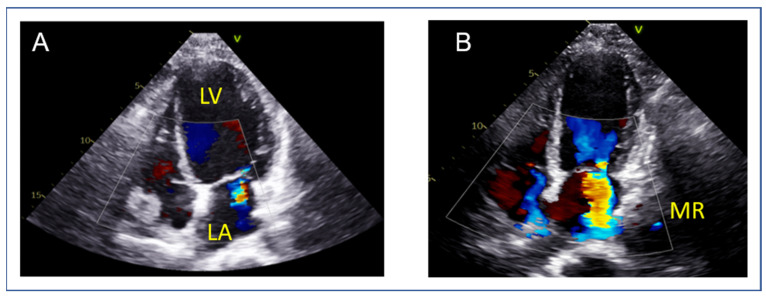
Ischemic dynamic MR unmasked by exercise echocardiography in a 66 y.o. patient with ischemic cardiomyopathy (LVEF = 25%) due to anterior infarct and exertional dyspnea NYHA III. (A) At rest, MR is mild, whereas (B) at 75 Watts the patient develops dyspnea upon aggravation of MR (EROA = 0.41 cm^2^, RVol = 39 mL) with significant elevation of pulmonary pressures (TR Vmax = 4.0 m/s, TR PeakPG = 65 mmHg, estimated SPAP = 70 mmHg). LA: left atrium, LV: left ventricle, MR: mitral regurgitation.
